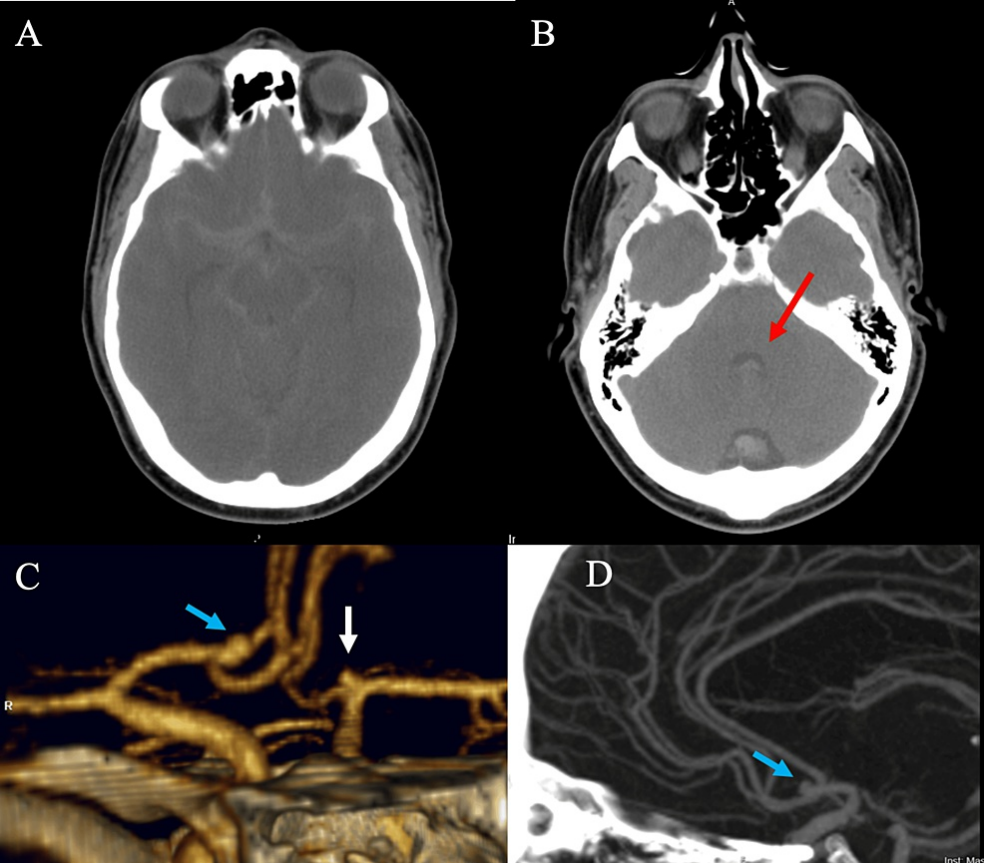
Computed tomographic images at presentation There was evidence of diffuse subarachnoid hemorrhage with extension to the Sylvian fissures, frontal sulci, cerebral hemispheres bilaterally (A), and intraventricular hemorrhage at the fourth ventricle (red arrow; B). CT angiography demonstrated a 3 mm right A1-A2 junction aneurysm, directed medially, anteriorly, and superiorly (blue arrow) and a 3 mm left bifurcation aneurysm (white arrow; C, D).
